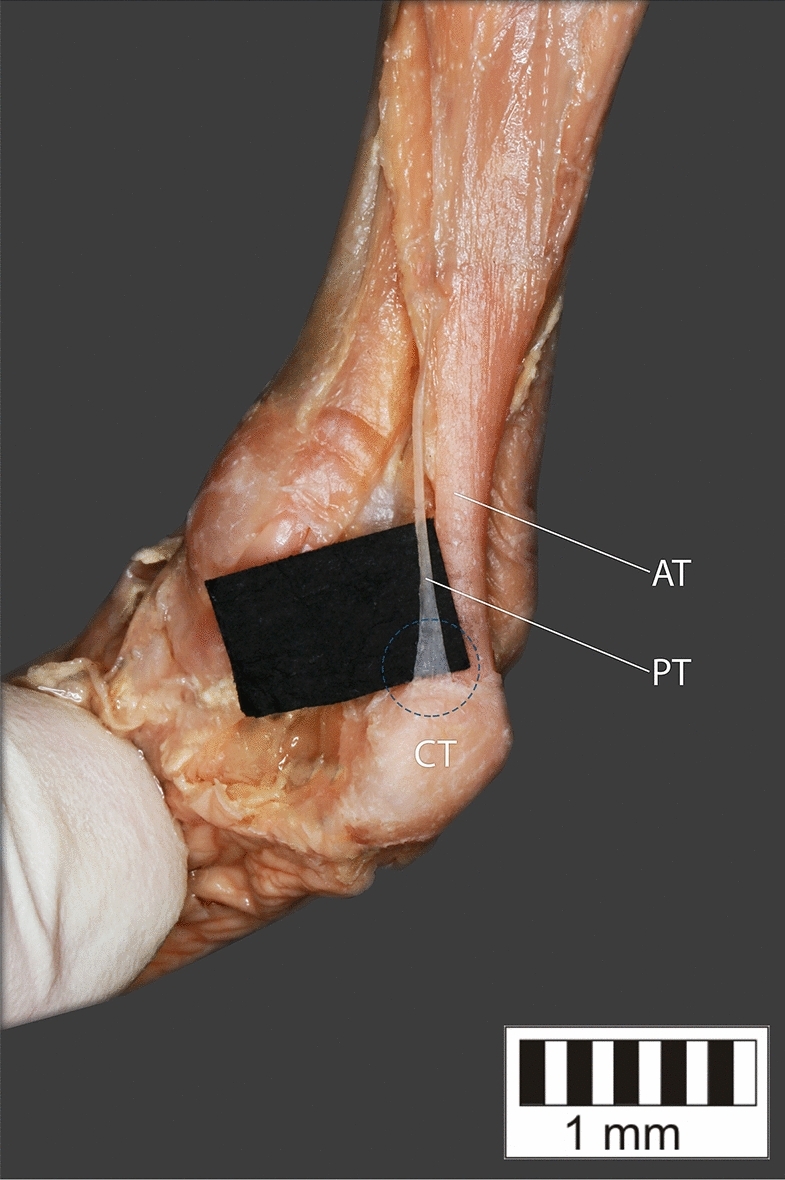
Type I of plantaris tendon. *CT *calcaneal tuberostity,* AT *Achilles tendon,* PT *plantaris tendon.
